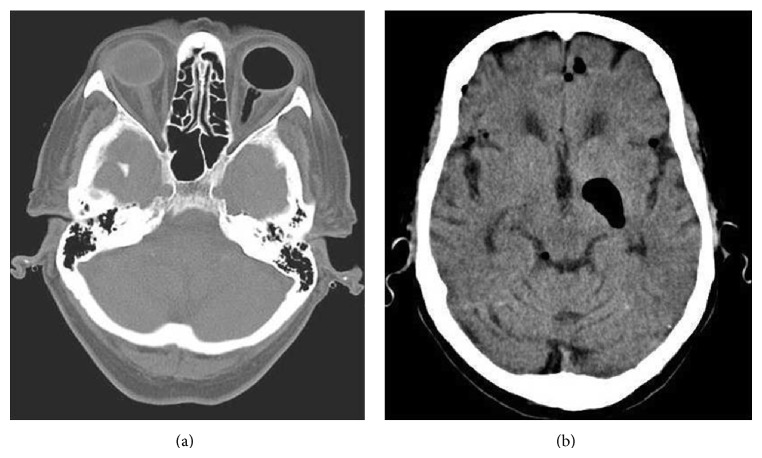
CT scan showing gas in the left eye and optic nerve (a) and intracranial air adjacent to the basal ganglia (b).
